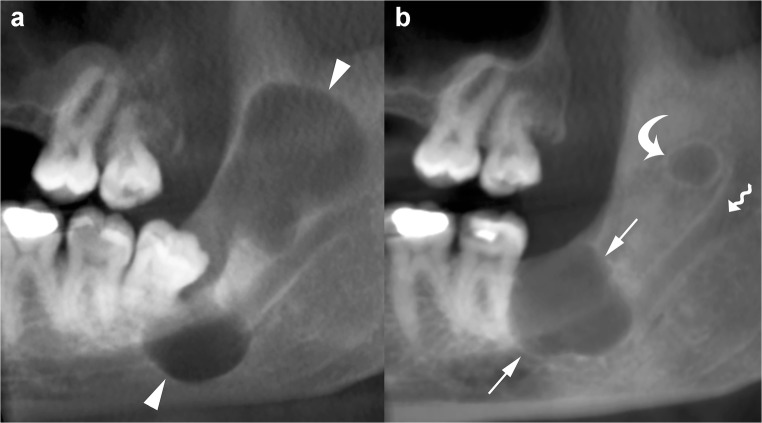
Panoramic CBCT images (20 mm thick) at baseline (a) and at the first follow-up (b). At baseline (a), a large unilocular OKC in the posterior mandible and ramus is shown (*arrowheads*). At the first follow-up (b), performed 2 years after surgery, the CBCT image shows two recurrences in the third molar region (*arrows*) and ramus of the mandible (*curved arrow*), respectively. *Wavy arrow*, mandibular foramen
